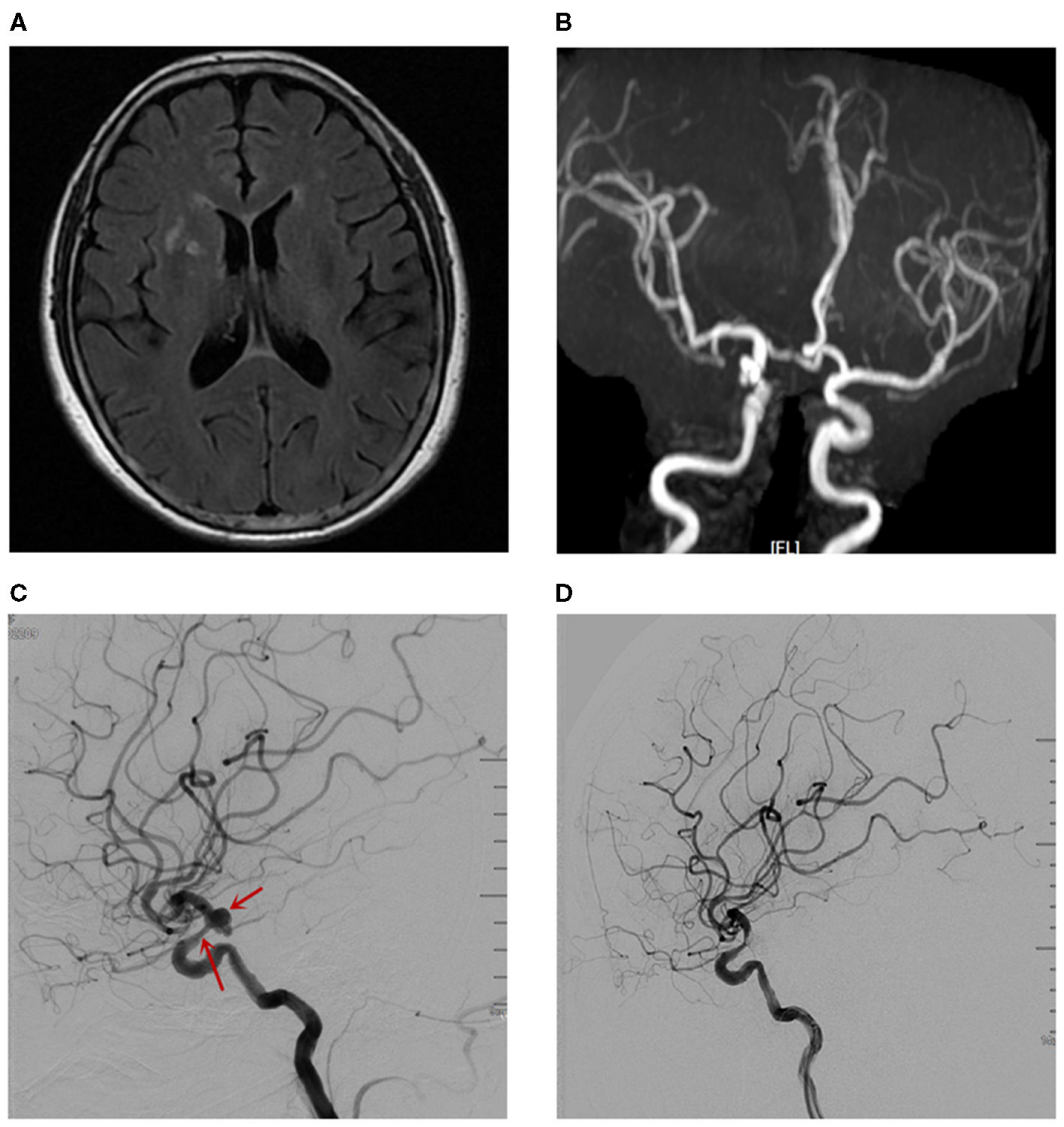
The imaging examinations before CIE. (A) MRI of the brain. (B) MRA detected the aneurysm in the communicating segment of the right ICA. (C) The DSA revealed an aneurysm with size of 7.4mm*6.1mm in the right ICA-communicating segment, and mild stenosis in right ICA-ophtalmic segment. (D) The DSA revealed a coiling embolization of the aneurysm.
